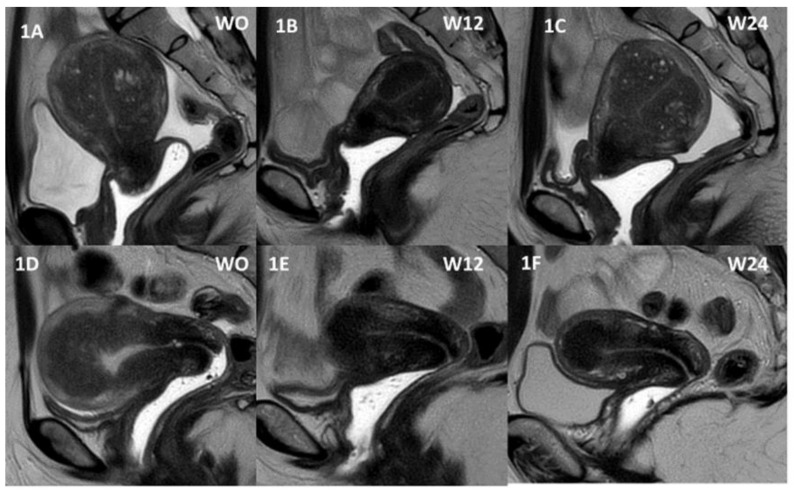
(A–C) Patient 1. (A) Magnetic resonance images showing an enlarged uterus with diffuse and disseminated adenomyosis at baseline. (B) After 12 weeks of GnRH antagonist therapy (daily dose 200 mg linzagolix), a significant reduction is observed in uterine size and adenomyotic lesions. (C) Patient was thereafter switched to linzagolix 100 mg/day, some regrowth was observed, but the volume remains significantly lower when compared to baseline. (D–F) Patient 2. MRI images at weeks 0, 12, and 24.
